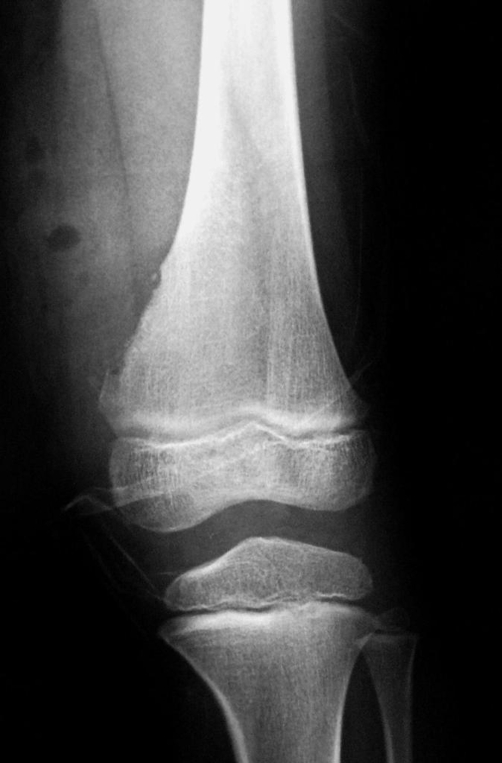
Antero-posterior plain radiographs of the femur immediately after the resection.
